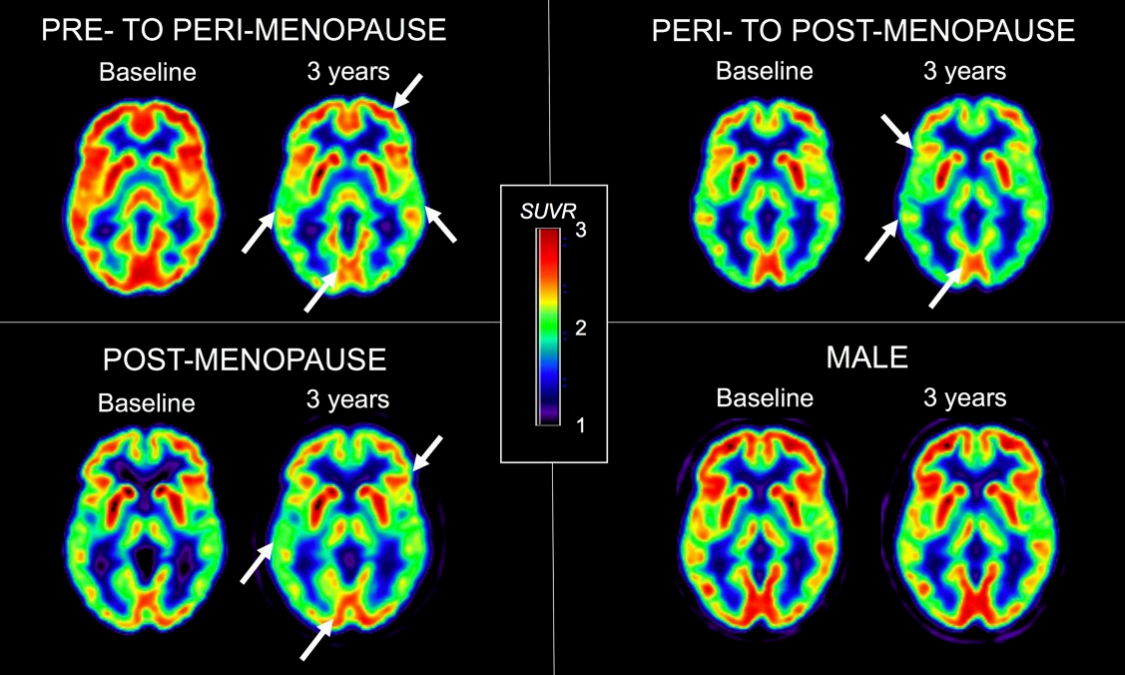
Baseline and follow-up FDG-PET scans in 4 representative cases. Top left: A 52 year-old woman (14 years education, APOE 3/4 carrier, CDR 0, MMSE 30) who was premenopausal at baseline and became perimenopausal by the follow-up exam. Top right: A 43 year-old woman (16 years education, APOE 3/3 carrier, CDR 0, MMSE 29) who was perimenopausal at baseline and became postmenopausal by the follow-up exam. Bottom left: A 59 year-old woman (16 years education, APOE 3/3 carrier, CDR 0, MMSE 30) who was postmenopausal at baseline. Bottom right: A 55 year-old man (14 years education, APOE 3/4 carrier, CDR 0, MMSE 30). Arrows point to areas of progressive CMRglc reductions in the follow-up vs. baseline scans. FDG measures are standardized uptake volume ratios (SUVR) adjusted for global uptake.
